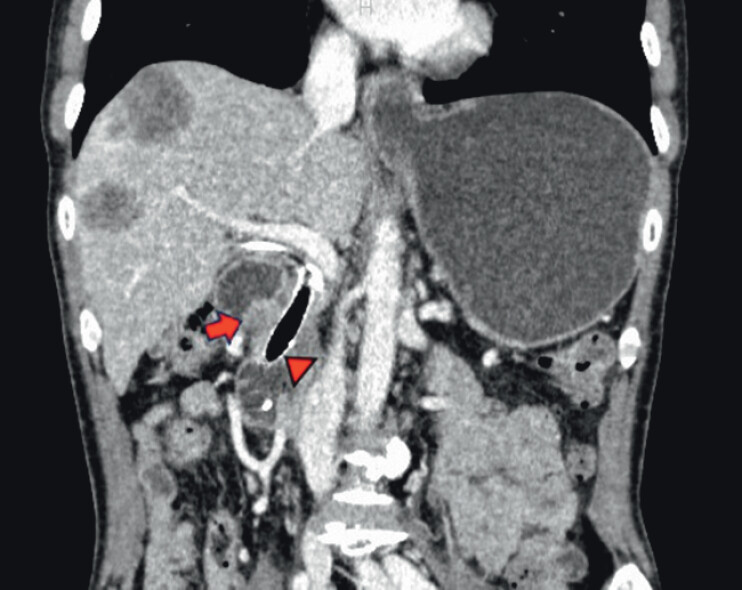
Contrast-enhanced computed tomography shows the malignant gastric outlet obstruction around the superior duodenal angle (arrow) caused by pancreatic head cancer. Arrow head, a biliary stent previously placed.
